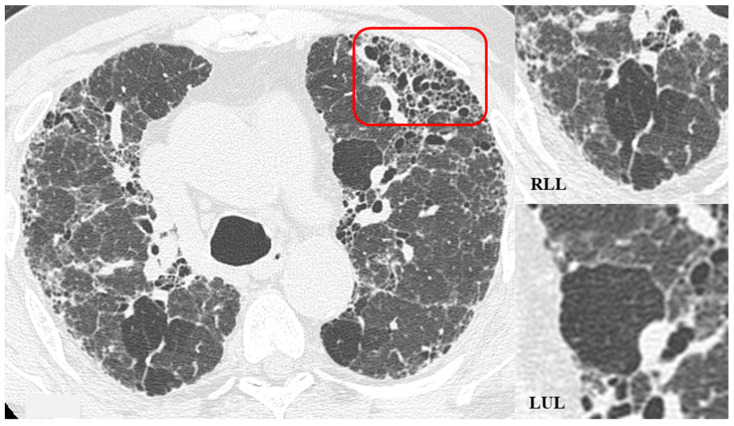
HRCT of the chest and magnification areas of RLL and LUL in FHP. Three-density pattern with lobular areas of reduced attenuation in both lungs (magnification images), overlapping with areas of increased density where distinct fibrotic alterations are evident, characterized by intra- and inter-lobular thickening with traction bronchiectasis and bronchiolectasis (red box). In certain patients, the absence of typical HRCT fibrotic features leads to their classification as “pattern compatible with FHP”. Suggestive elements of this pattern are fibrosis with a mixed central and peripheral distribution (Figure 7) and the fibrotic pattern with basal predominance (Figure 8).
